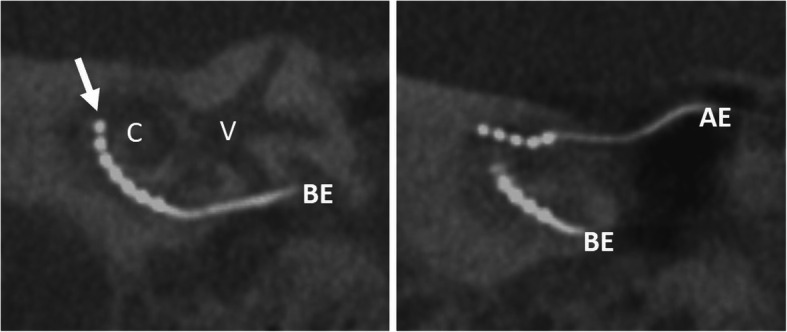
A 70-year-old male patient, with normal postoperative finding after split electrode surgery due to far advanced otosclerosis. CBCT paracoronal image (left) shows basal electrode (BE) with the tip up to the first half of the basal turn (arrow). CBCT paraaxial maximum intensity projection image shows the additional apical electrode (AE) in the middle turn. C – cochlea. V – vestibulum
